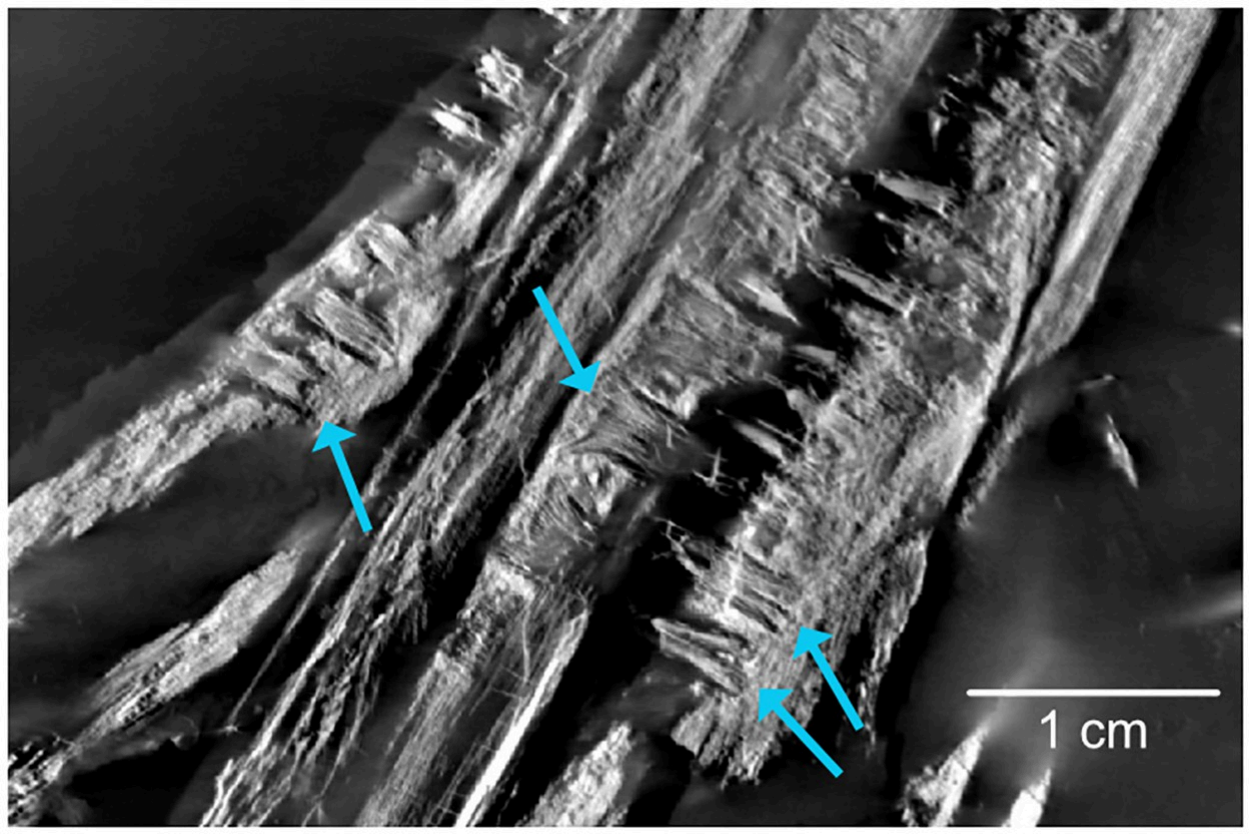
Detailed radiograph of the dentition of PMO 219.250. Blue arrows indicate where grooves in the tooth roots can be observed.
